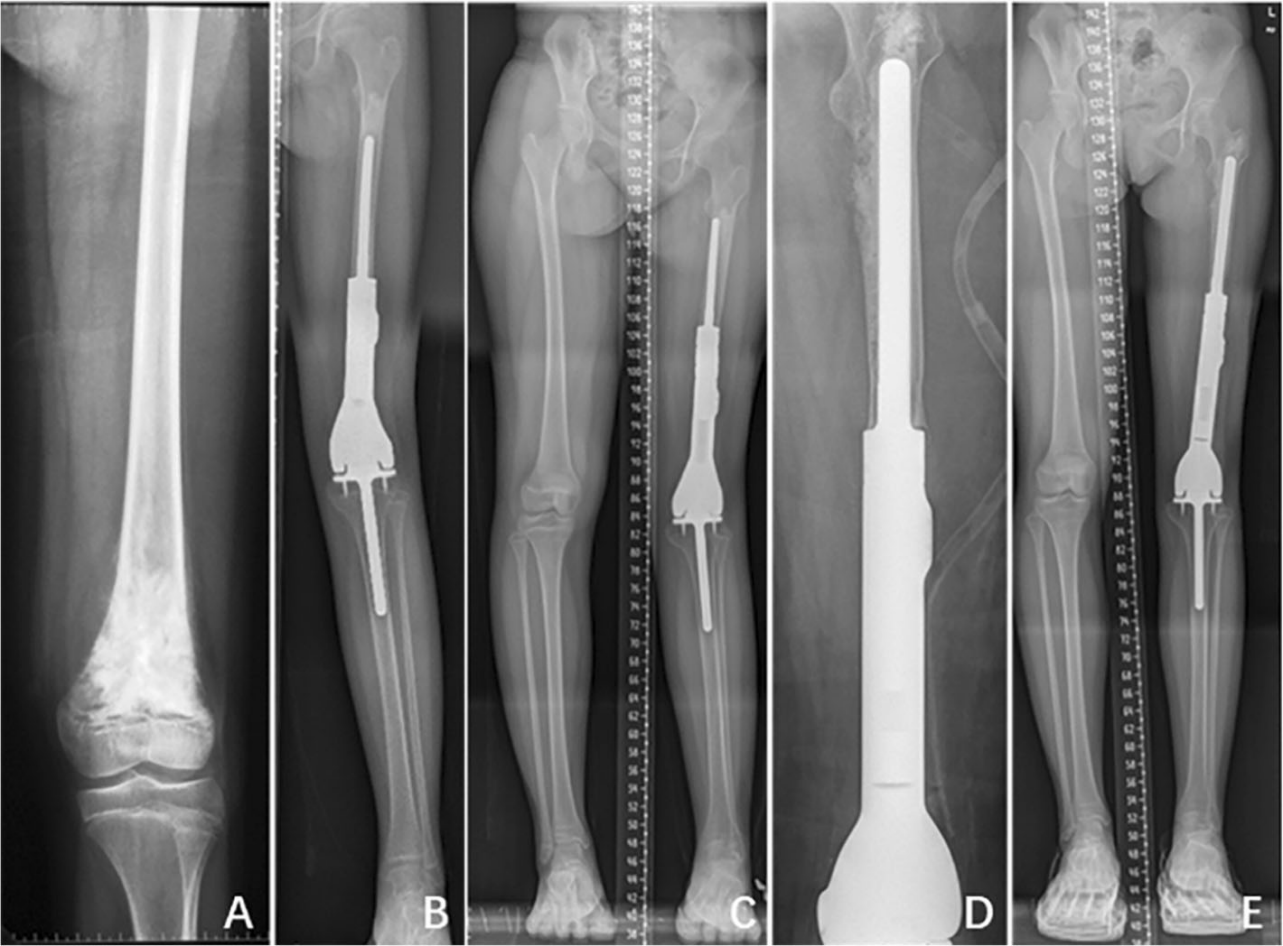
The lengthening and revision of a 9-year-old girl with osteosarcoma in the left distal femur. a X-ray indicates osteosarcoma in the left distal femur. b The patient underwent tumor resection and implantation of extendible prosthesis. c Prosthesis displacement and out of length after extension of 4 cm in 2 years. d The revision operation was performed, and a new prosthesis with longer and thicker stem was implanted. f The LLDs were less than 1 cm after another 2 cm extension
